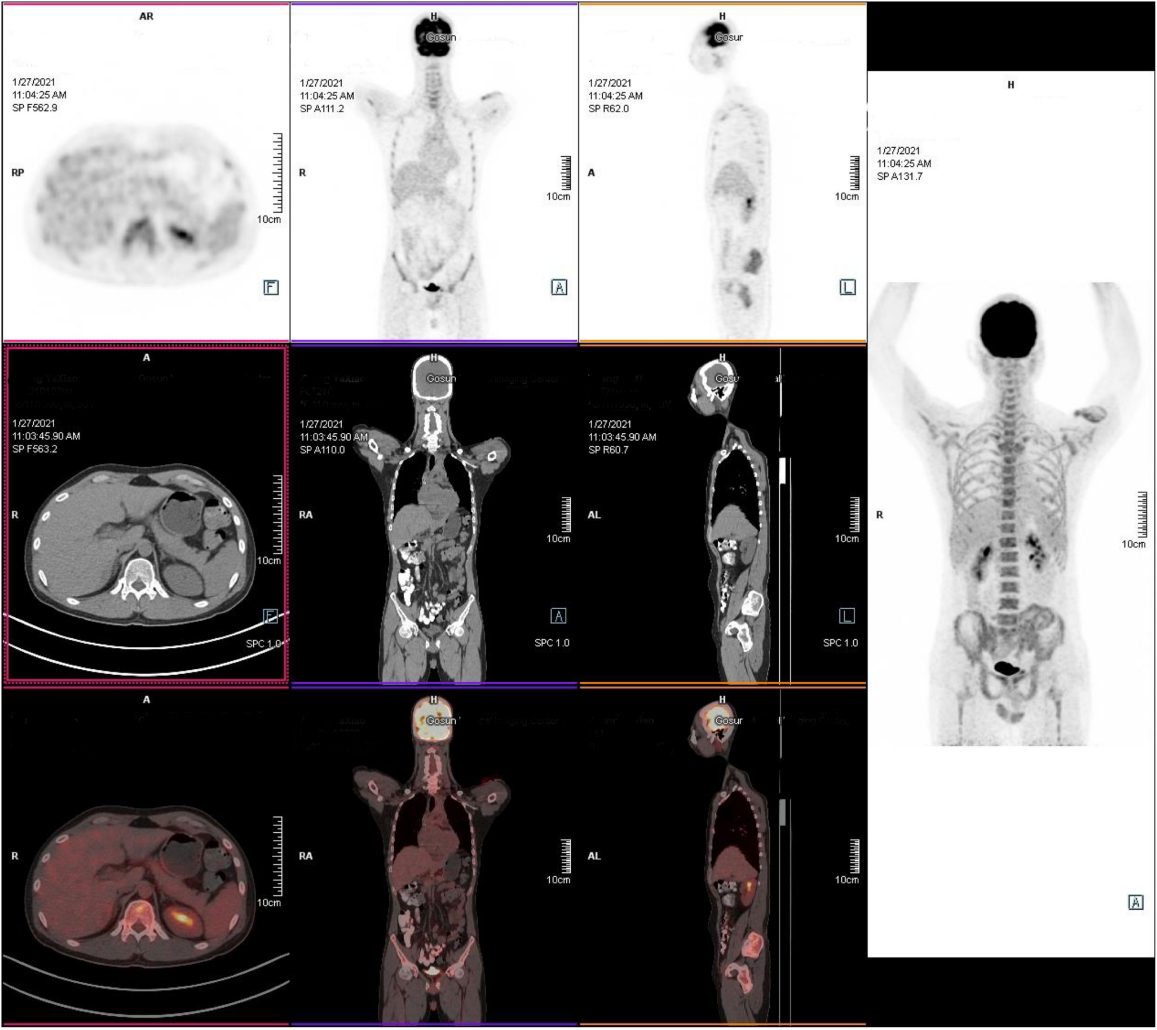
Positron emission tomography/computed tomography examination. The tumor was in remission without radiographic recurrence. (1) There was no increase in lesion metabolism in the original mediastinal, neck, axillar, supraclavicular, and internal mammary areas; the Deauville score was 1, which was considered complete remission after treatment. (2) Left upper-arm and lower-left abdominal-wall skin and subcutaneous inflammatory changes.
